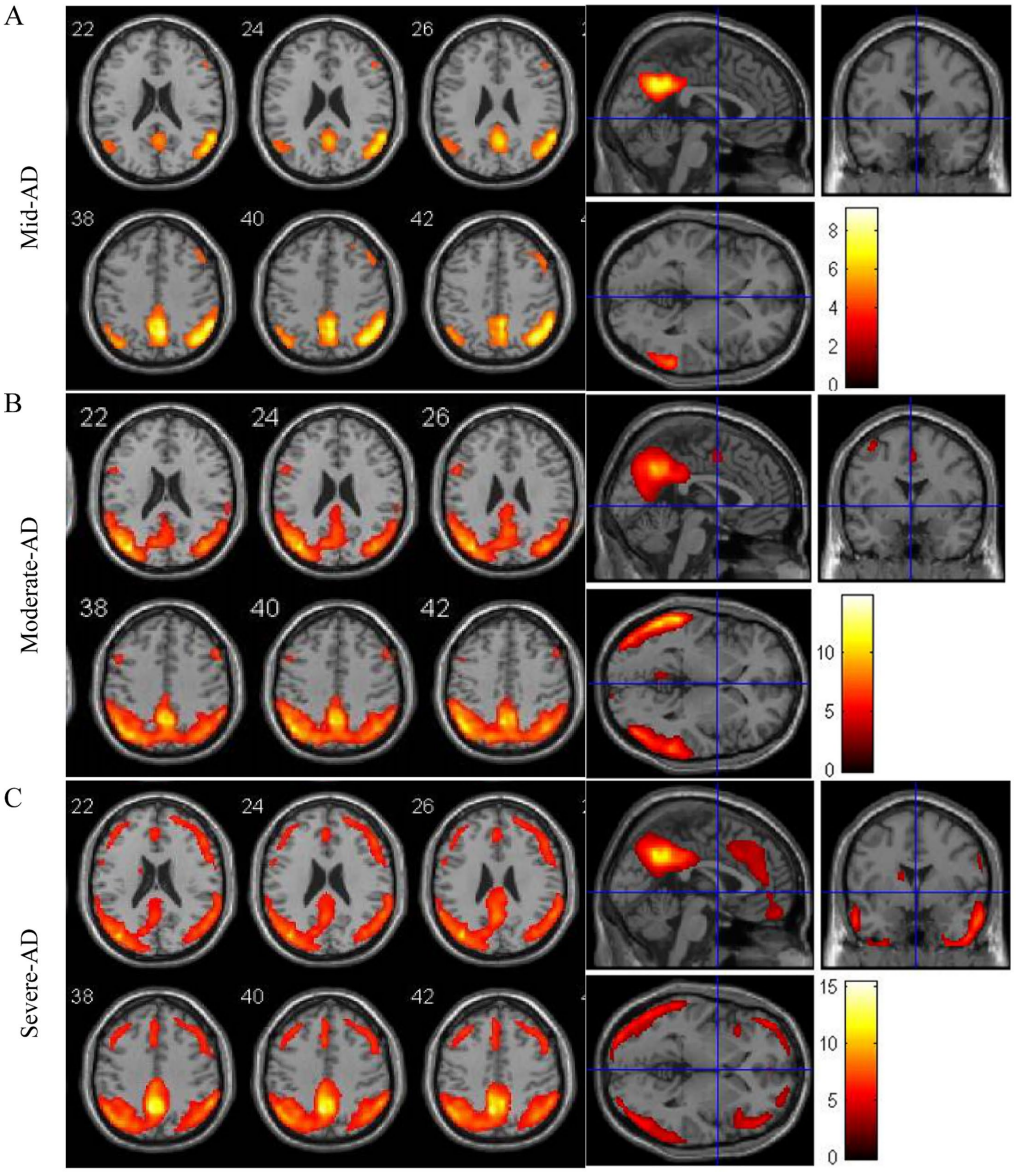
Atrophy maps of AD disease with different degrees of progression analyzed by ^18^FDG-PET-CT. (A–C) Typical image of AD characterized by atrophy of the temporo-parietal cortex.
